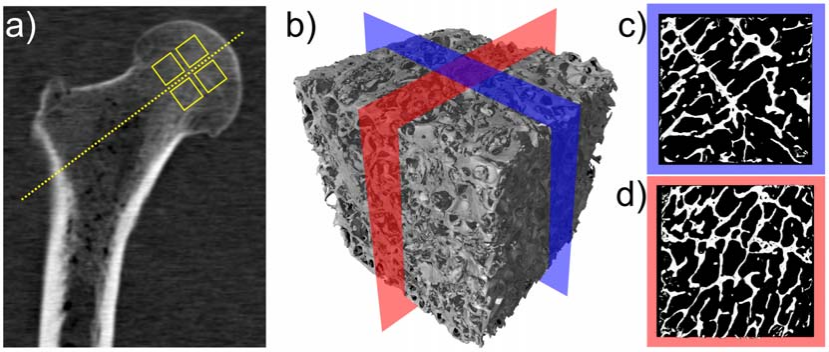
(a) Slice of a clinical CT of the upper part of an entire femur bone. The samples used in this study were prepared from the region around the rotational axis of the femoral head. This location is indicated by the four boxes. (b) Three-dimensional surface rendering of a high resolution *μ*CT of a 1 cm^3^ cube of human femur bone sample P7. (c,d) Two-dimensional cuts (10 mm × 10 mm) through both horizontal axes, taken from the centre region as indicated in (b).
